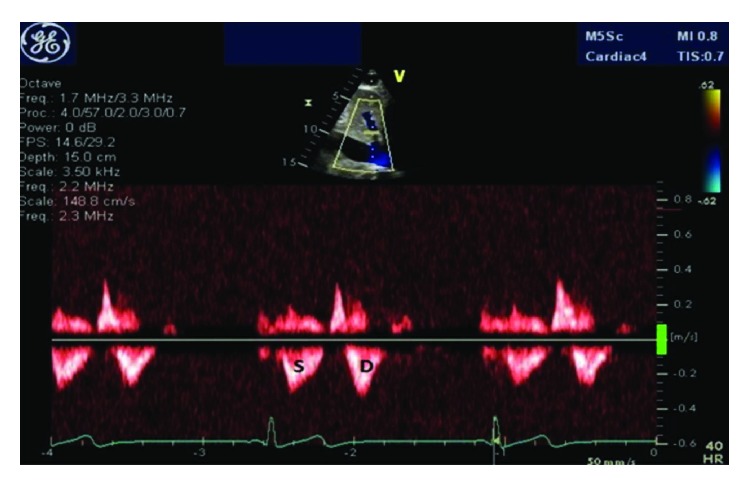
Hepatic flow Doppler. Doppler signal marked S for systolic and D for diastolic. Informal visualization shows systolic velocity (Vs) of 0.32 m/sec and diastolic velocity (Vd) of 0.38 m/sec.
